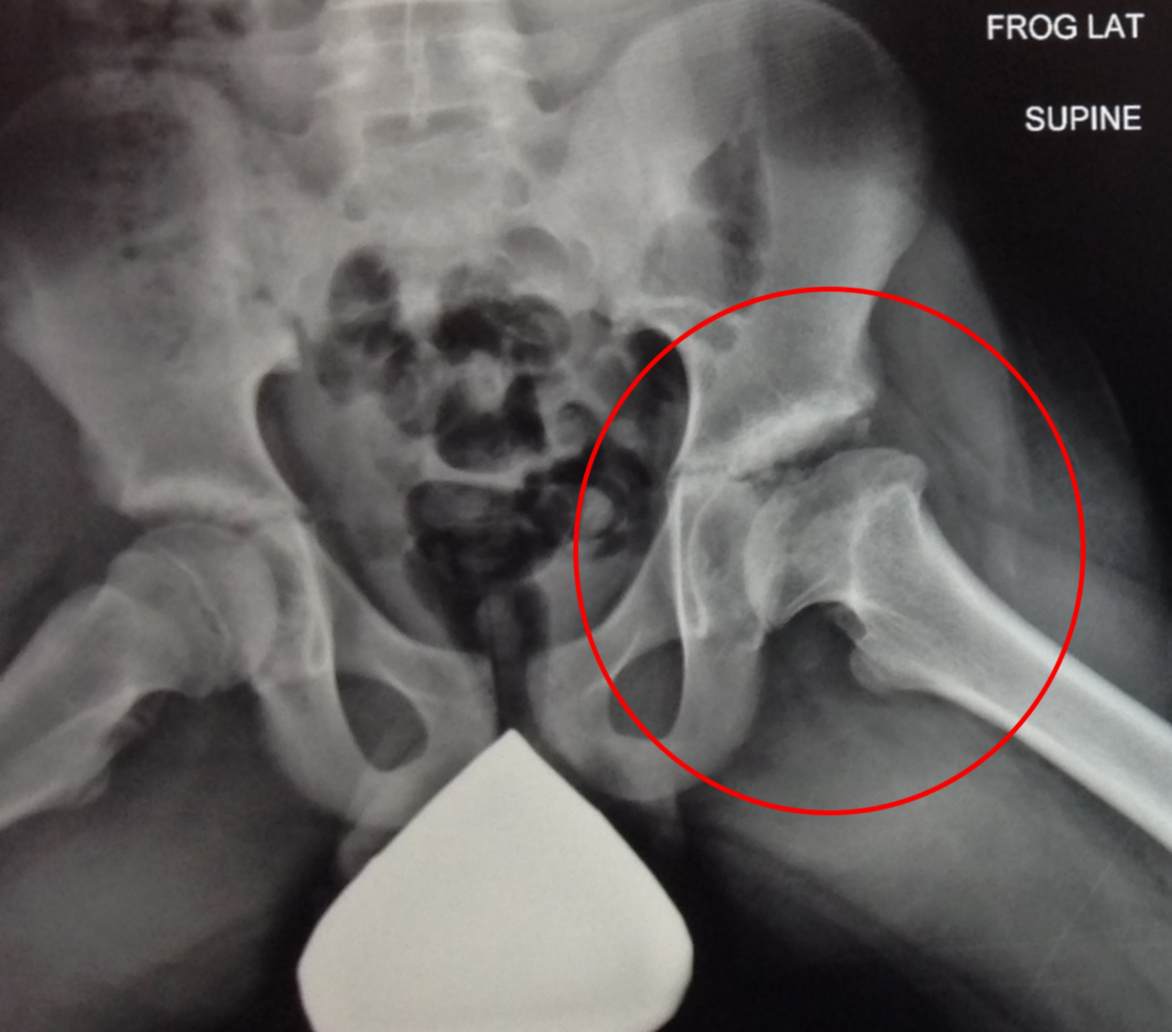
Frog leg lateral view pelvic X-ray taken on January 6, 2016.
